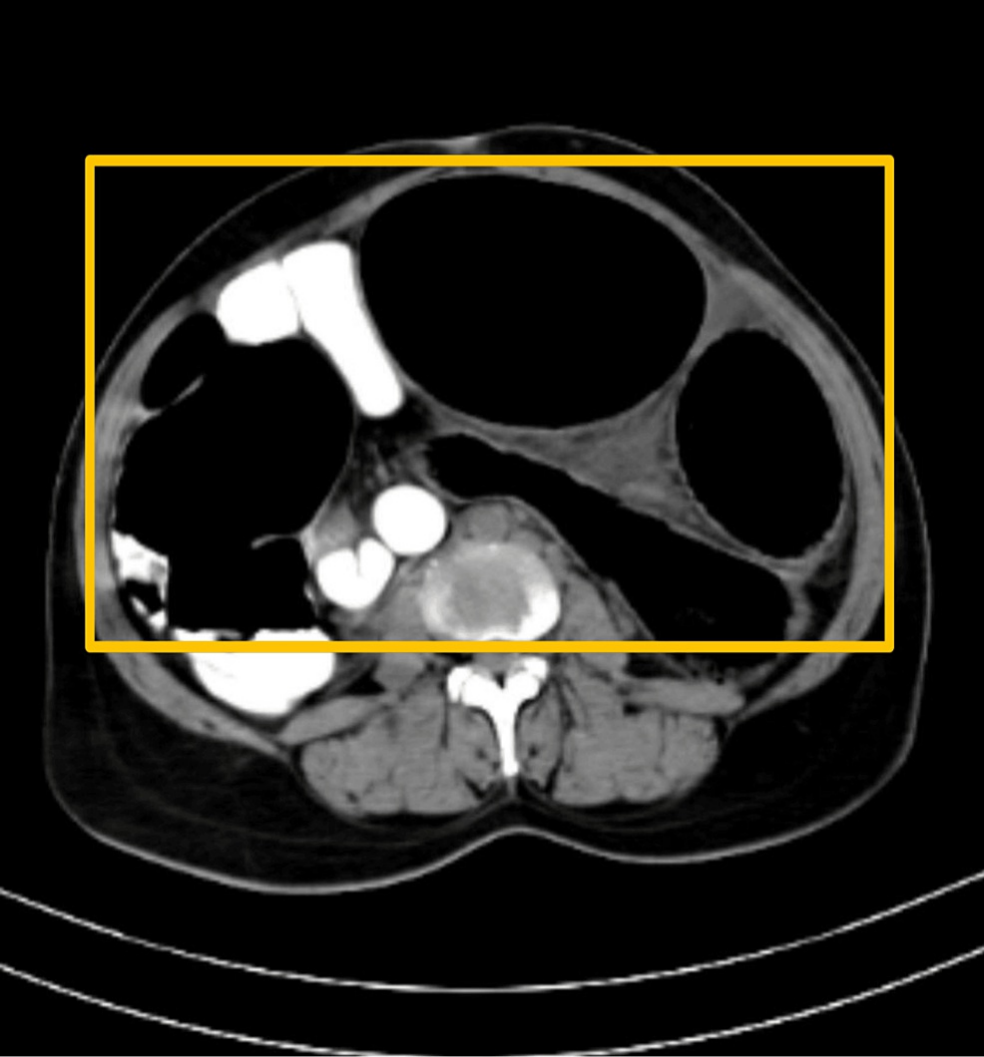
CT of the abdomen showing a dilated sigmoid loop likely to be sigmoid volvulus
